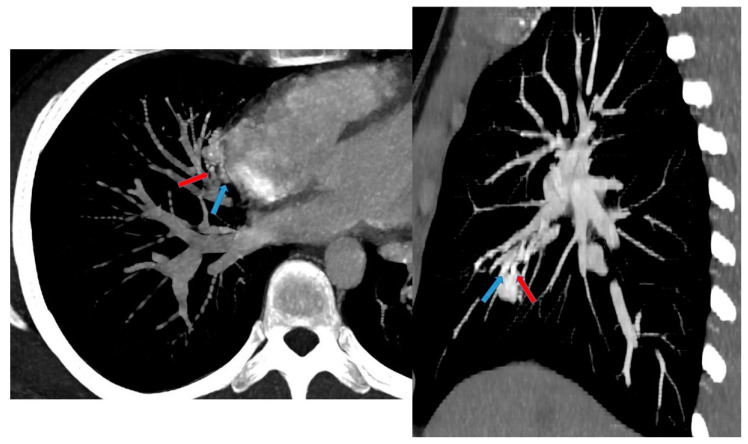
A 17 year old patient with hereditary hemorrhagic telangiectasis (HHT). CT angiography with maximum intensity projection (MIP) reconstruction shows a lobulated enhancing lesion in the right middle lobe, with a feeding artery (red arrow) and a draining vein (blue arrow), compatible with pulmonary arteriovenous malformation.
